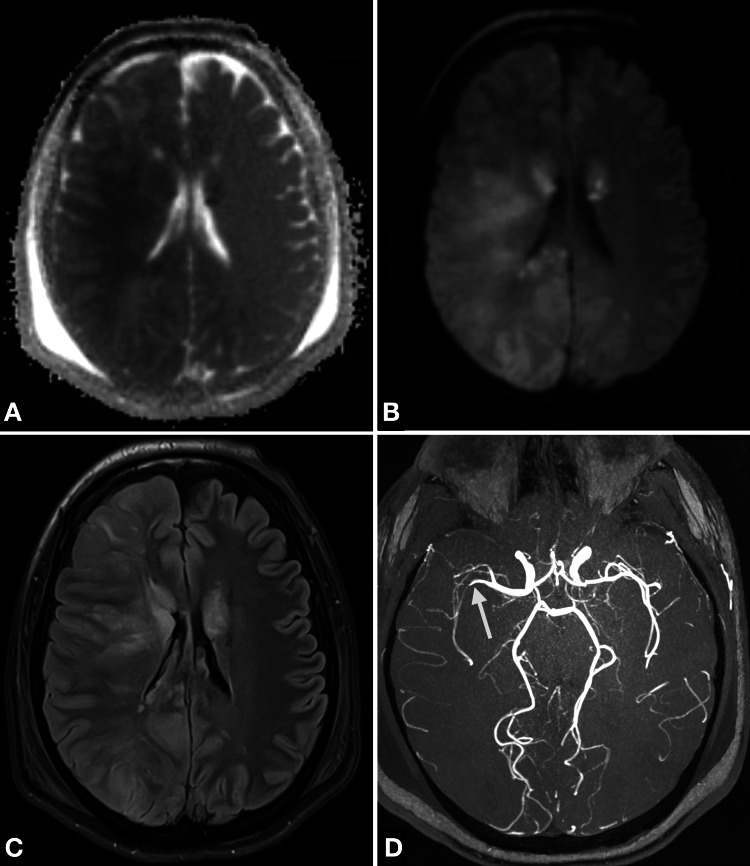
Brain magnetic resonance imaging for prognostic evaluation (A) Apparent diffusion coefficient and (B) Diffusion-weighted imaging (b1000) demonstrate bilateral supratentorial diffusion restriction, most pronounced in the right parieto-occipitotemporal region and basal ganglia. (C) Fluid-attenuated inversion recovery sequence demonstrating hyperintense signals corresponding to the areas of diffusion restriction. (D) Magnetic resonance angiography showing residual vasospasms with calibre irregularities in the right middle cerebral artery and both the anterior cerebral arteries (arrows).
